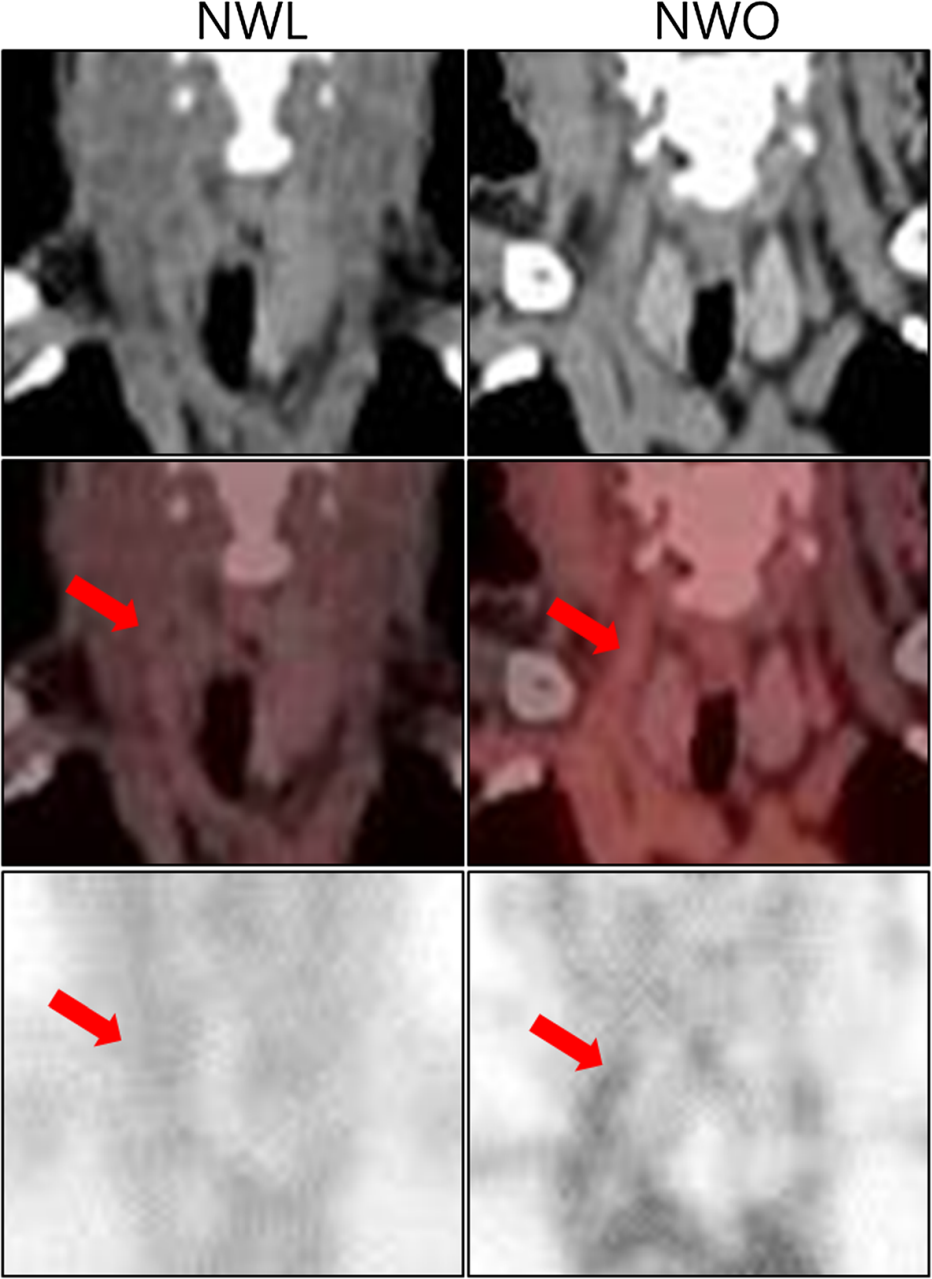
Representative figures indicating the SUV uptake at the carotid artery in NWL and NWO subjects. Coronal image of ^18^ F-FDG-PET/CT around the carotid artery is presented as an unenhanced CT image, a fusion image of CT and PET, and a maximum intensity projection (MIP) image, from the upper panel to the lower panel. The red arrow indicates the carotid artery. ^18^ F-FDG-PET/CT, ^18^ F-fludeoxyglucose positron emission tomography/computed tomography; SUV, standardized uptake value; NWL, normal weight lean; NWO, normal weight obesity.
